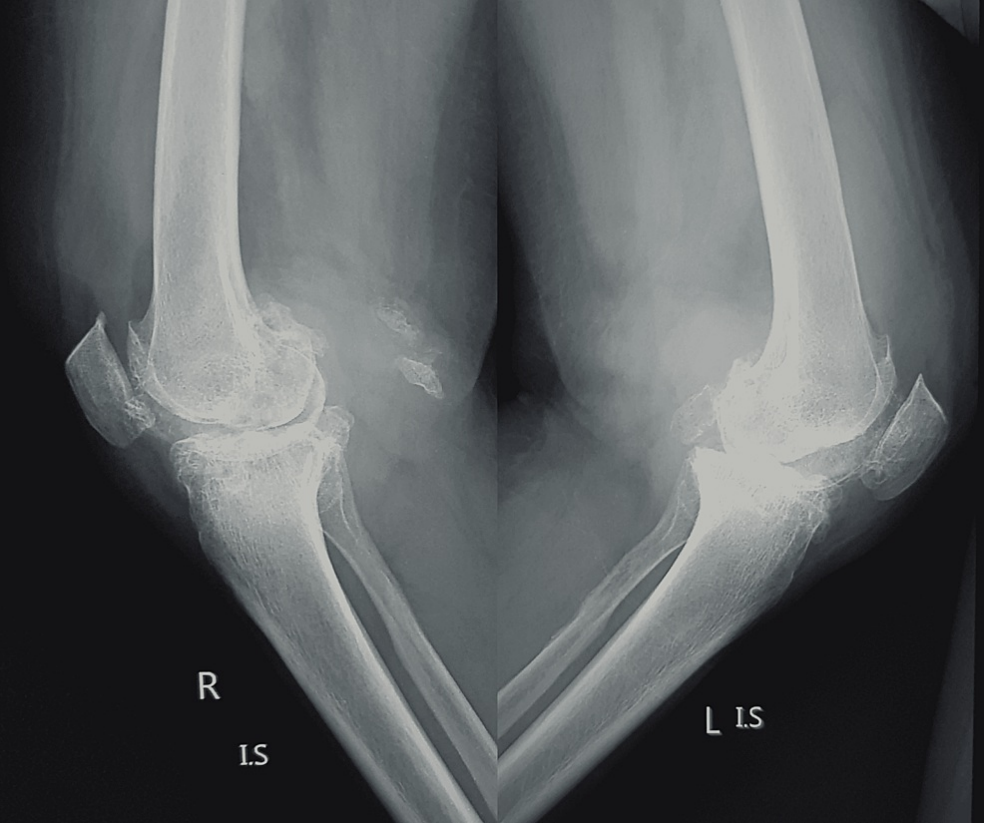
Lateral view radiograph of the bilateral knee joint with severe osteoarthritis.
